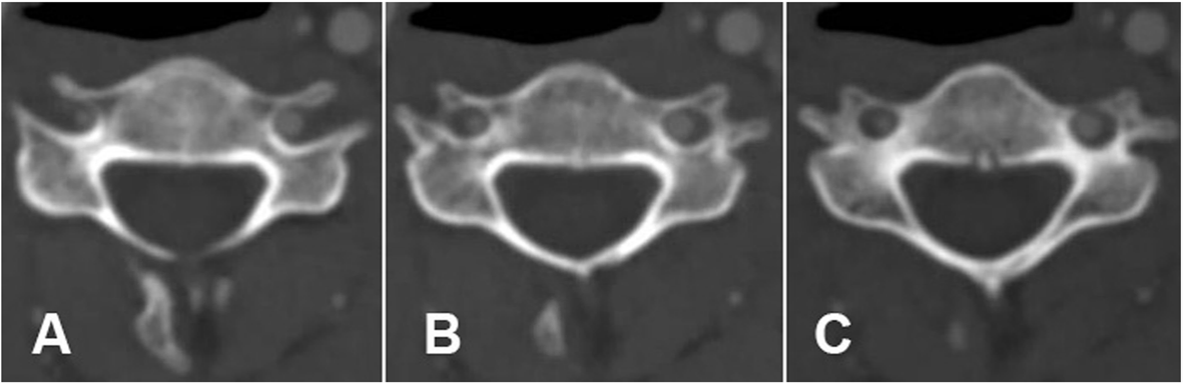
Series CT images of C4 show that the patient with VAD exhibit smaller POW on the dominant side than that on the non-dominant side (A: 4.43 mm VS 5.41 mm; B: 4.92 mm VS 5.73 mm; C: 4.42 mm VS 5.34 mm; Averaged POW: 4.59 mm VS 5.49 mm). VAD, vertebral artery dominance; POW, the distance between the lateral cortical border and medial border at the most stenotic area of the pedicle
